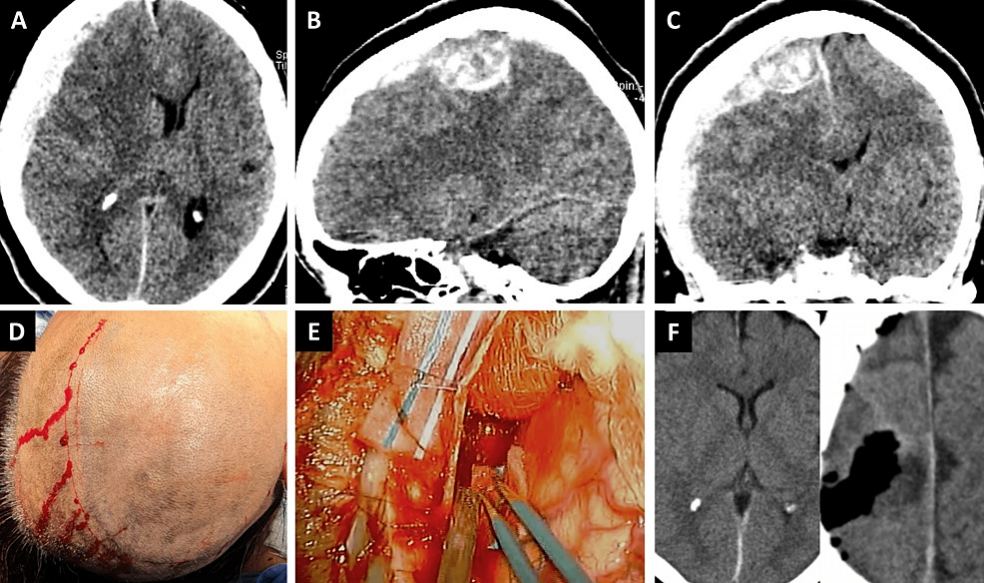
CT studies and view of the surgical approach. A preoperative CT study (axial view) demonstrates a right-sided subdural hemorrhage over the frontal lobe (A) and over the parietal lobe (sagittal view) (B). A midline shift is accompanied by the collapse and compression of the third and right lateral ventricles (C). Falconer’s approach was performed with subsequent frontoparietal-temporal craniotomy (D). The hematoma was drained, and the tumor was excised (E). Postoperative CT demonstrates reversal of the midline shift and meningioma resection (F).
